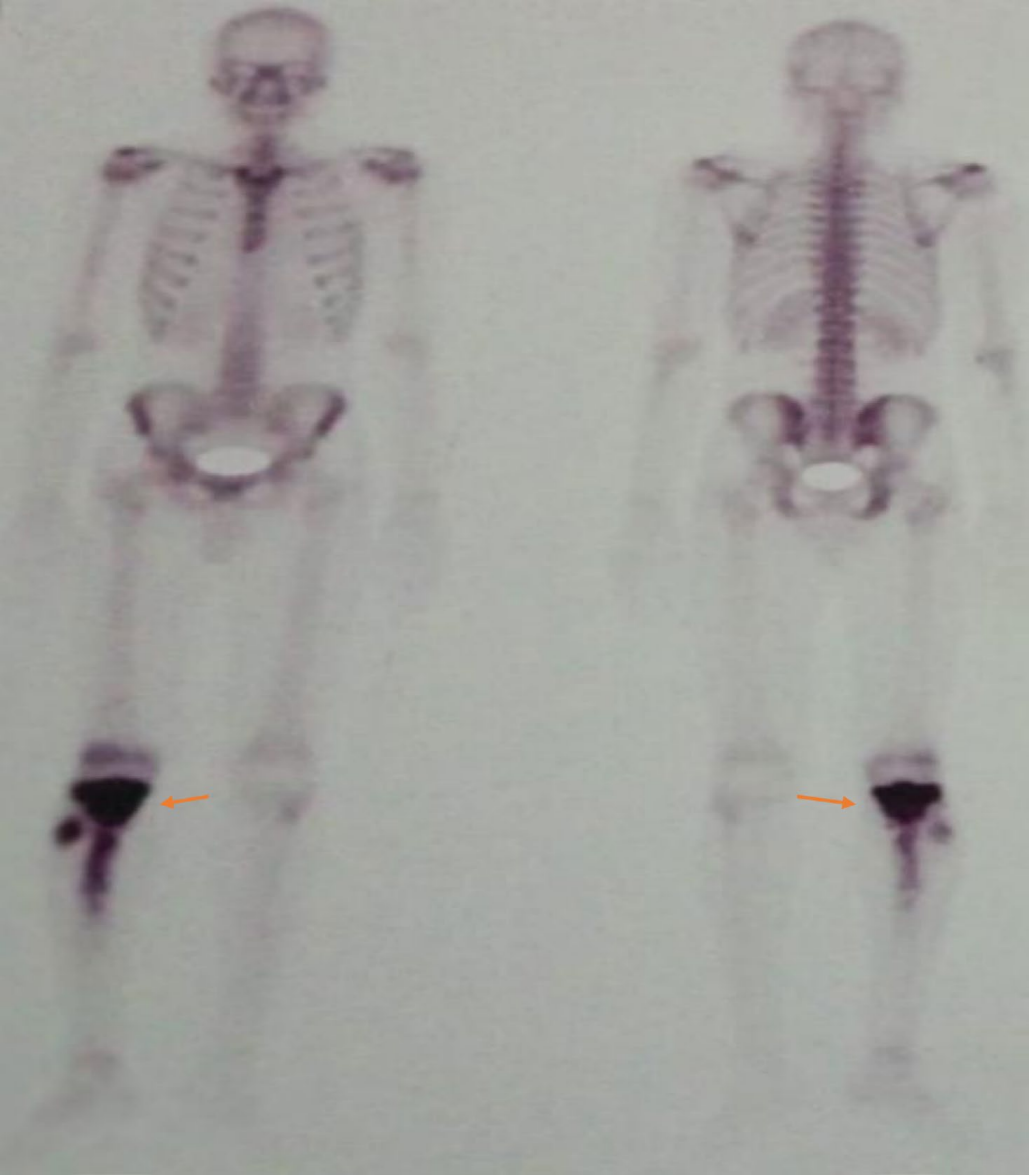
Initial staging with Technetium‐99m bone scintigraphy. Anterior whole‐body bone scan demonstrates intense, heterogeneous radiotracer uptake localized to the metaphyseal‐diaphyseal region of the right proximal tibia (arrow), consistent with the primary chondroblastic osteosarcoma. The scan revealed no evidence of synchronous skeletal metastases at the time of diagnosis.
